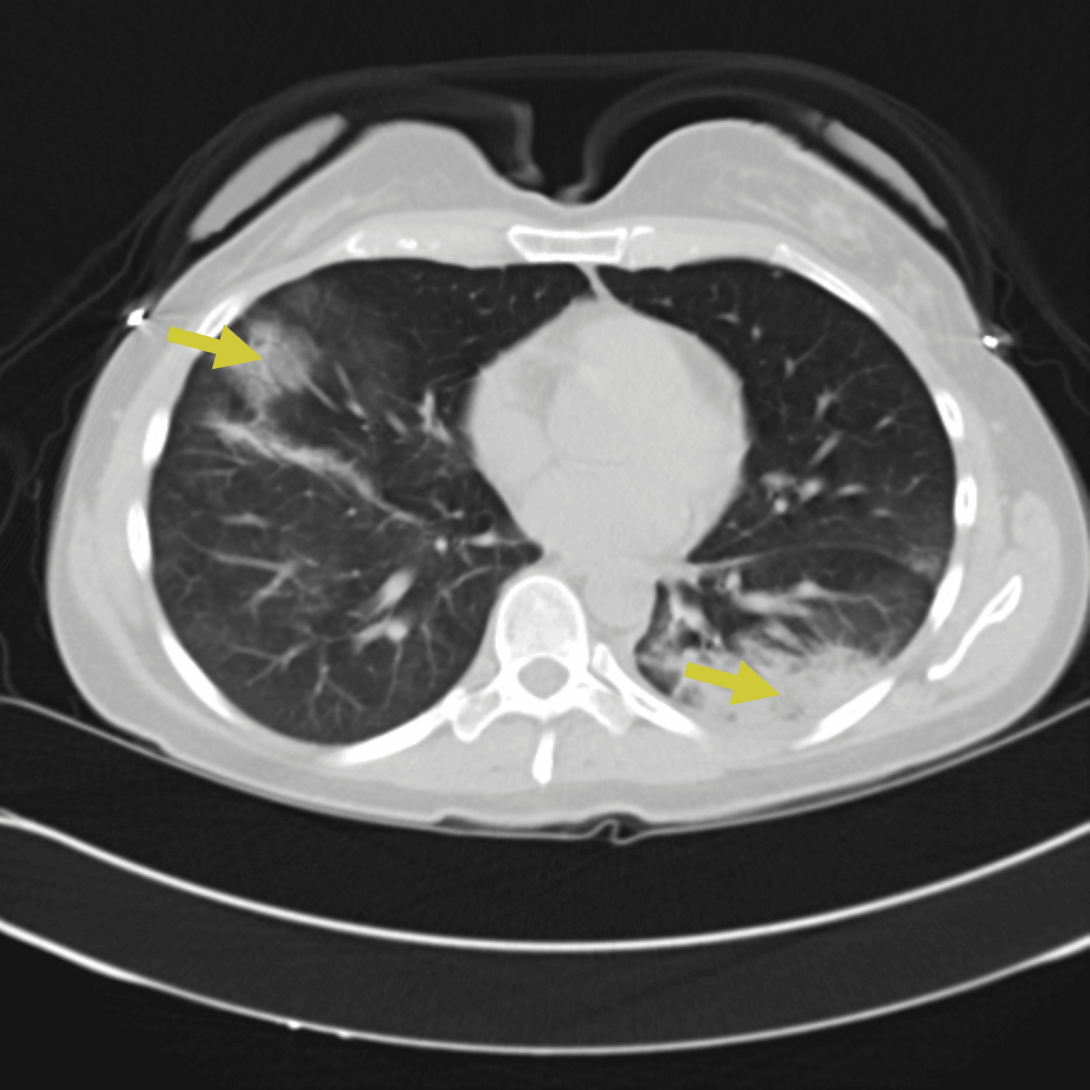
CT chest obtained on presentation showing bilateral ground-glass opacities and consolidations (yellow arrows)
